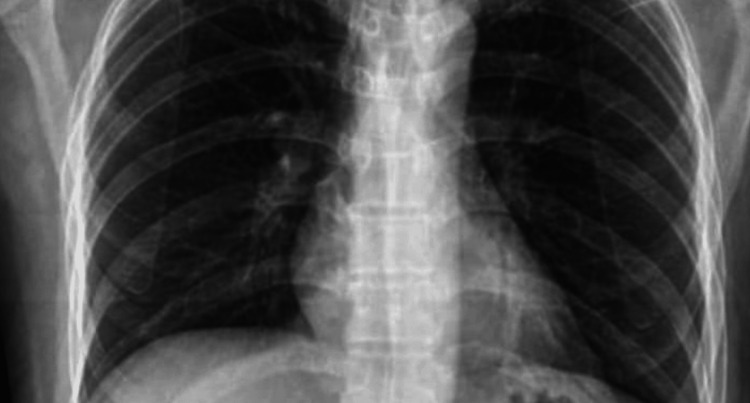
Normal chest X-ray (PA view)
